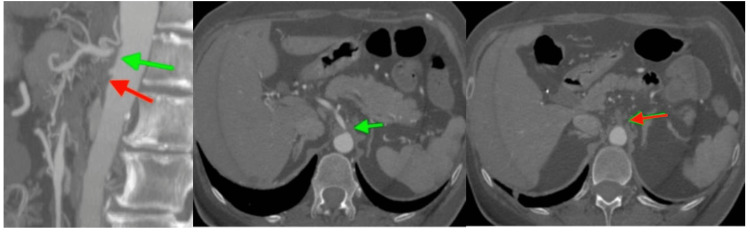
CTA of the abdominal aorta, showing ostial occlusion of the coeliac artery (green arrow) and occlusion of the SMA at its origin (red arrow). CTA, computed tomography angiography; SMA, superior mesenteric artery
